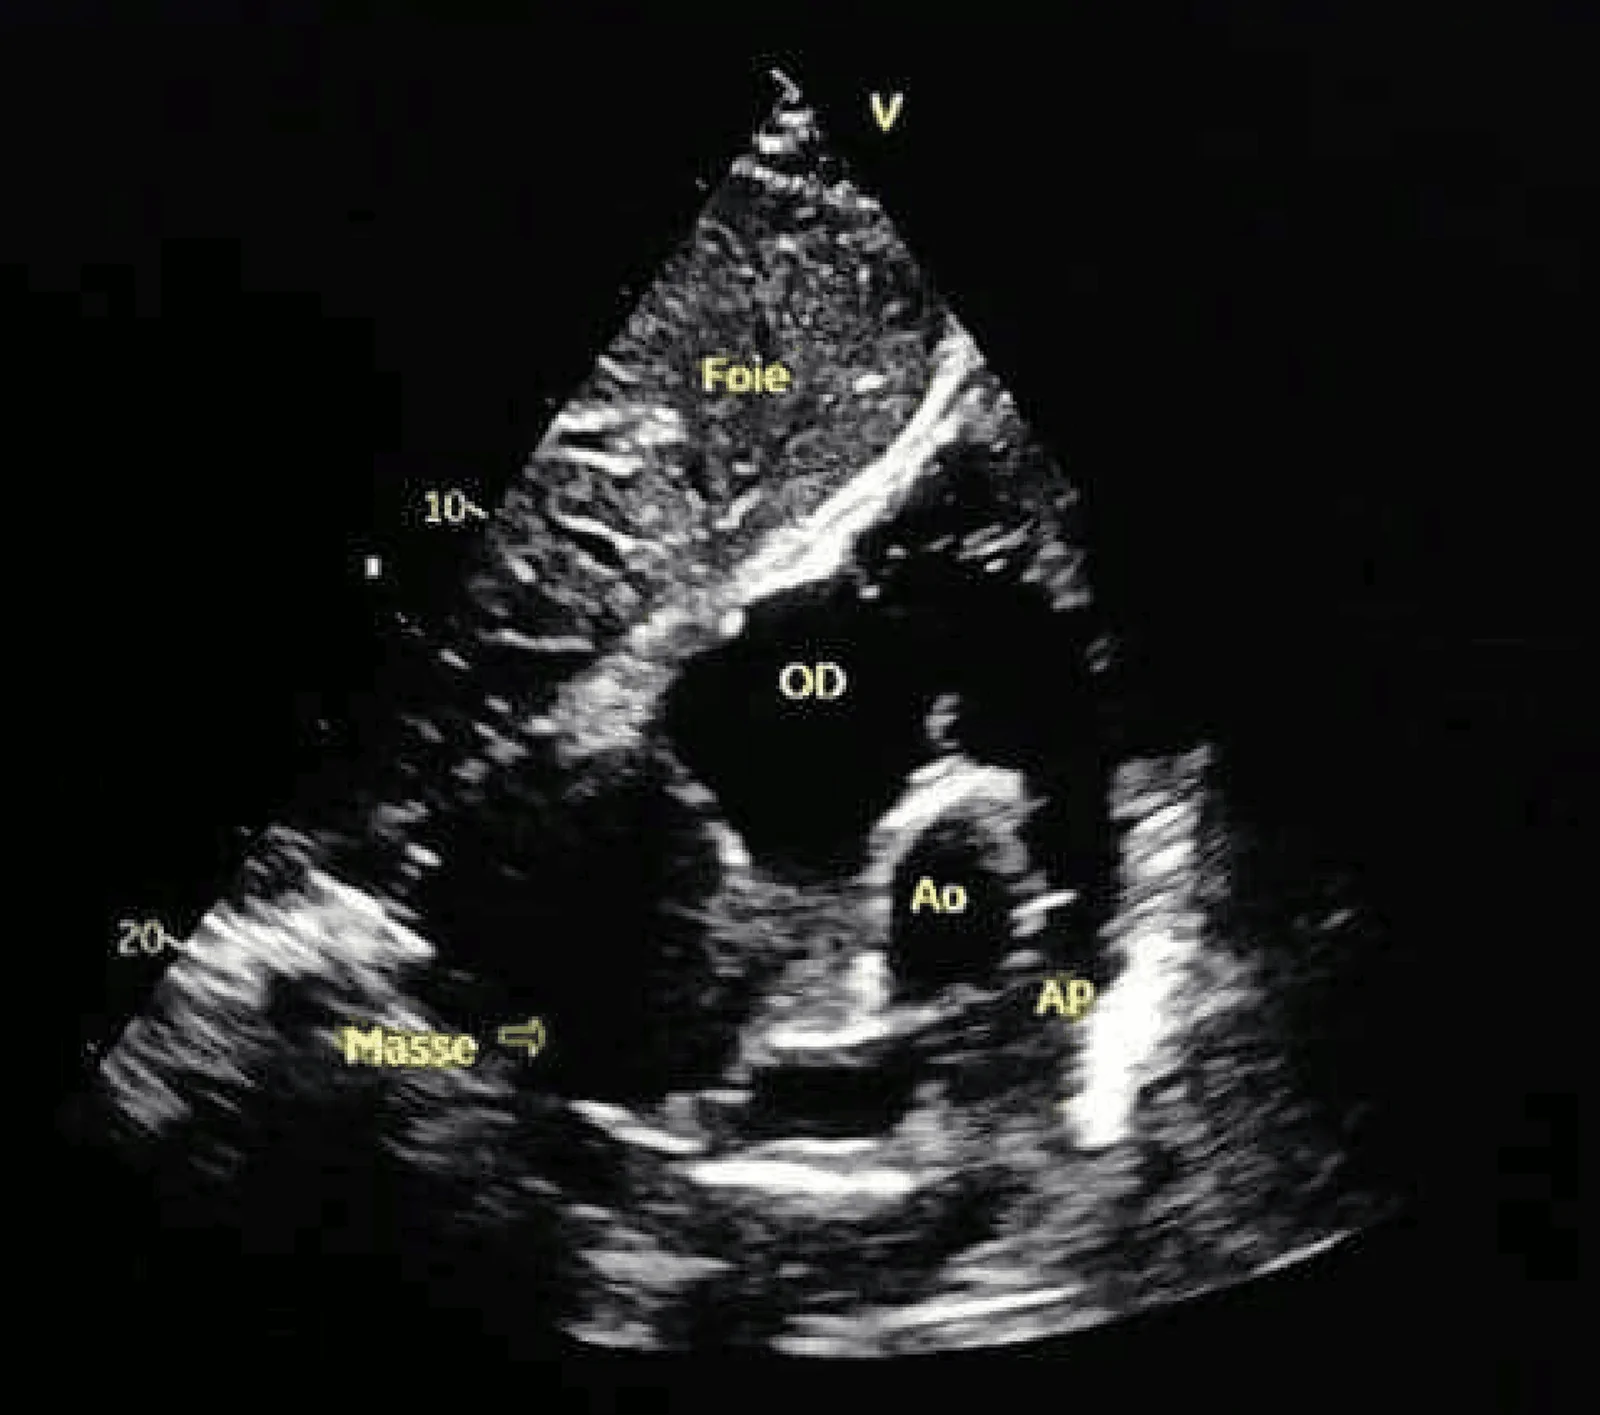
Subcostal echocardiographic view revealing a large mediastinal mass compressing the cardiac structures OD: *l'oreillette droite* (right atrium); OD: *l'oreillette droite* (right atrium); AO: aorta; AP: *artère pulmonaire* (pulmonary artery); *foie*: liver; *Masse*: mass (Original labels are in French)
